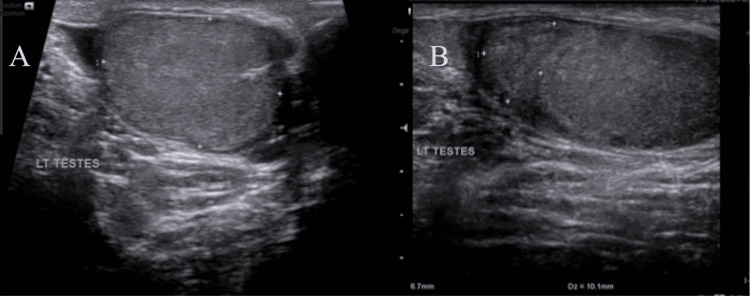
Initial testicular ultrasound of the left testis that revealed a homogeneous testis with an area of decreased parenchymal echogenicity
